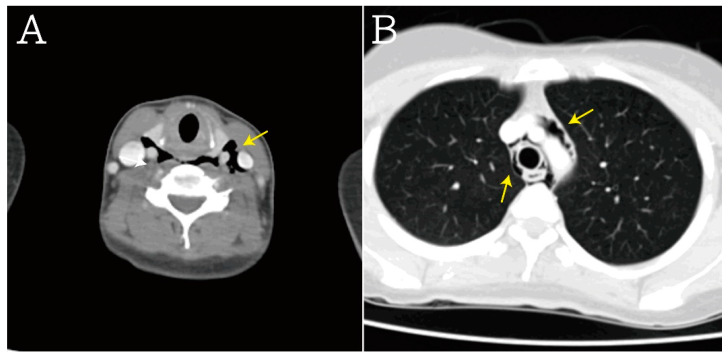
In computed tomography scans, these results were also noted, including (A) subcutaneous emphysema (yellow arrow), (B) pneumomediastinum, periaortic gas, and pericardiac gas (yellow arrow).
